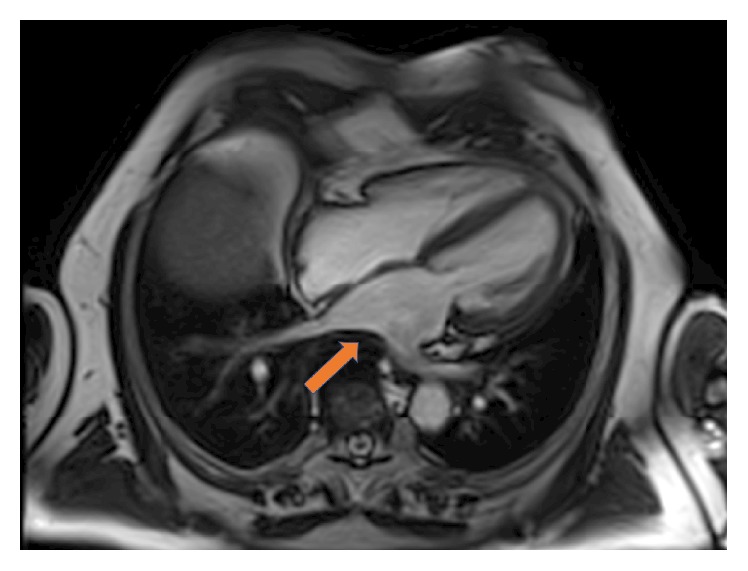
Magnetic resonance image of the heart showing the osteophyte protruding into the left atrium.
